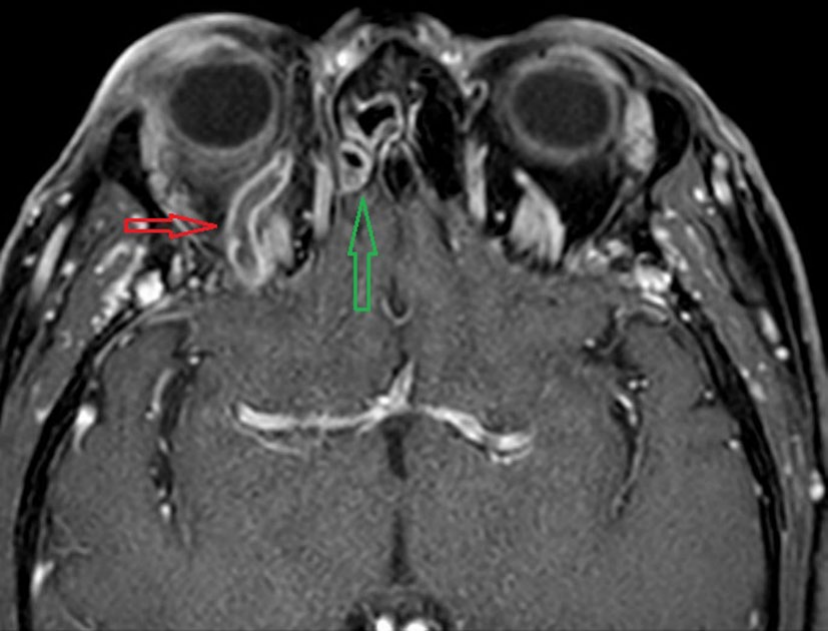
Contrast-enhanced MRI (3D T1GE; E-THRIVE) dilated right superior ophthalmic vein with thrombosis (red arrow) and the enhancement in the right ethmoid sinus (green arrow)
